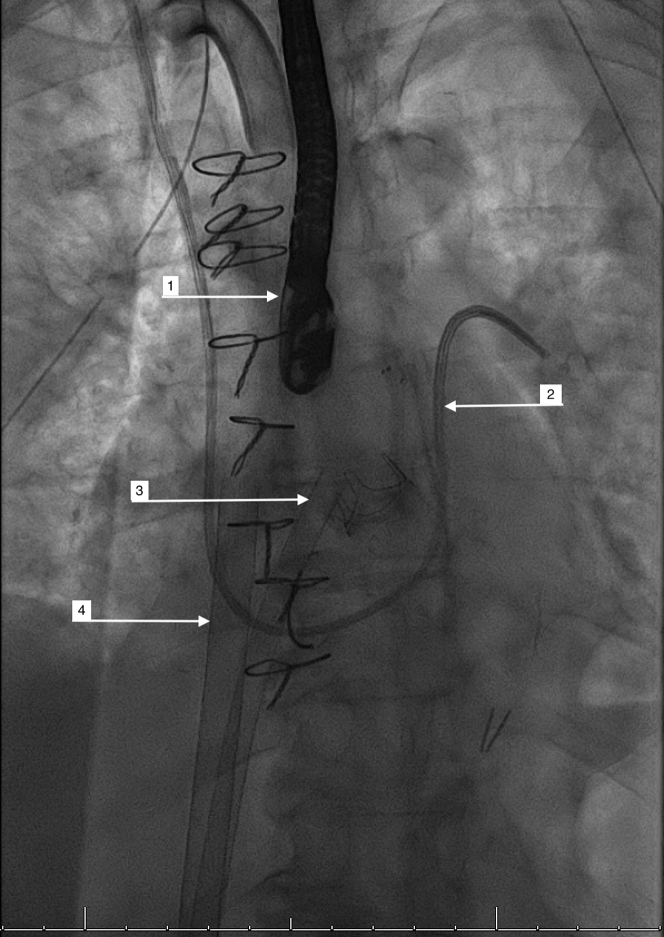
Fluoroscopic Image of Venous-Left Atrial Extracorporeal Membrane Oxygenation configuration Cannula Placement Arrows represent (1) transesophageal echocardiography probe, (2) Swan-Ganz catheter, (3) left atrial cannula, and (4) venous cannula.
